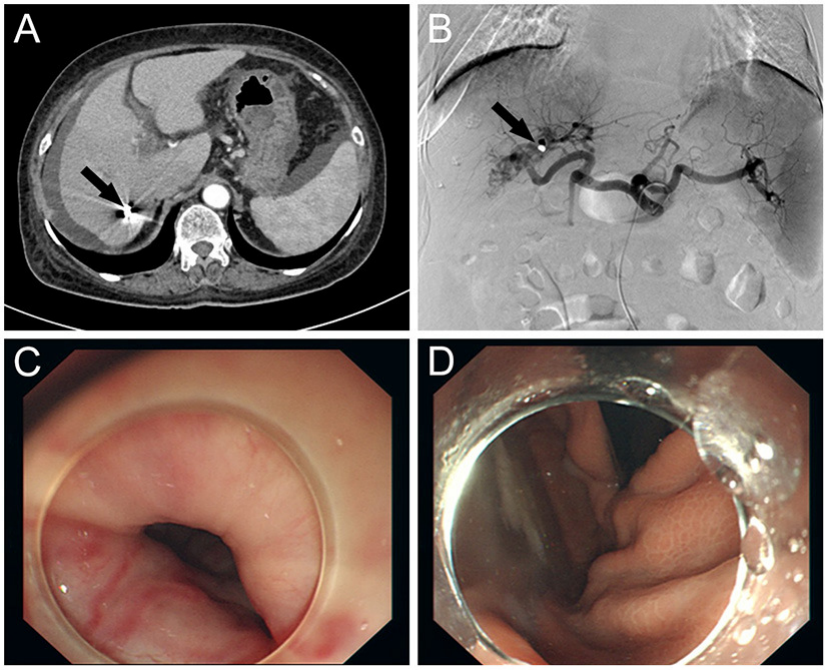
(A) CT scan of the abdomen: CT shows occlusion of the hepatic arterioportal fistula after embolization (black arrow) and reduction of ascites. (B) Selective hepatic artery angiography showing complete closure of the fistula (black arrow) between the right hepatic artery and the right portal vein branch after coil embolization. (C) Improvement of varicose veins after endoscopic sclerotherapy. (D) Improvement of varicose veins after endoscopic sclerotherapy.
